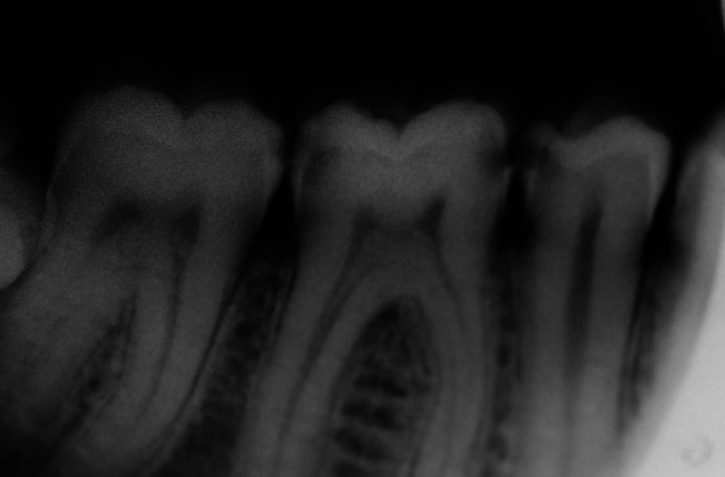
Radiographic image of the clinical case evaluated in the survey. The posterior sector of the fourth quadrant can be seen. (44, 45, 46 and 47).
